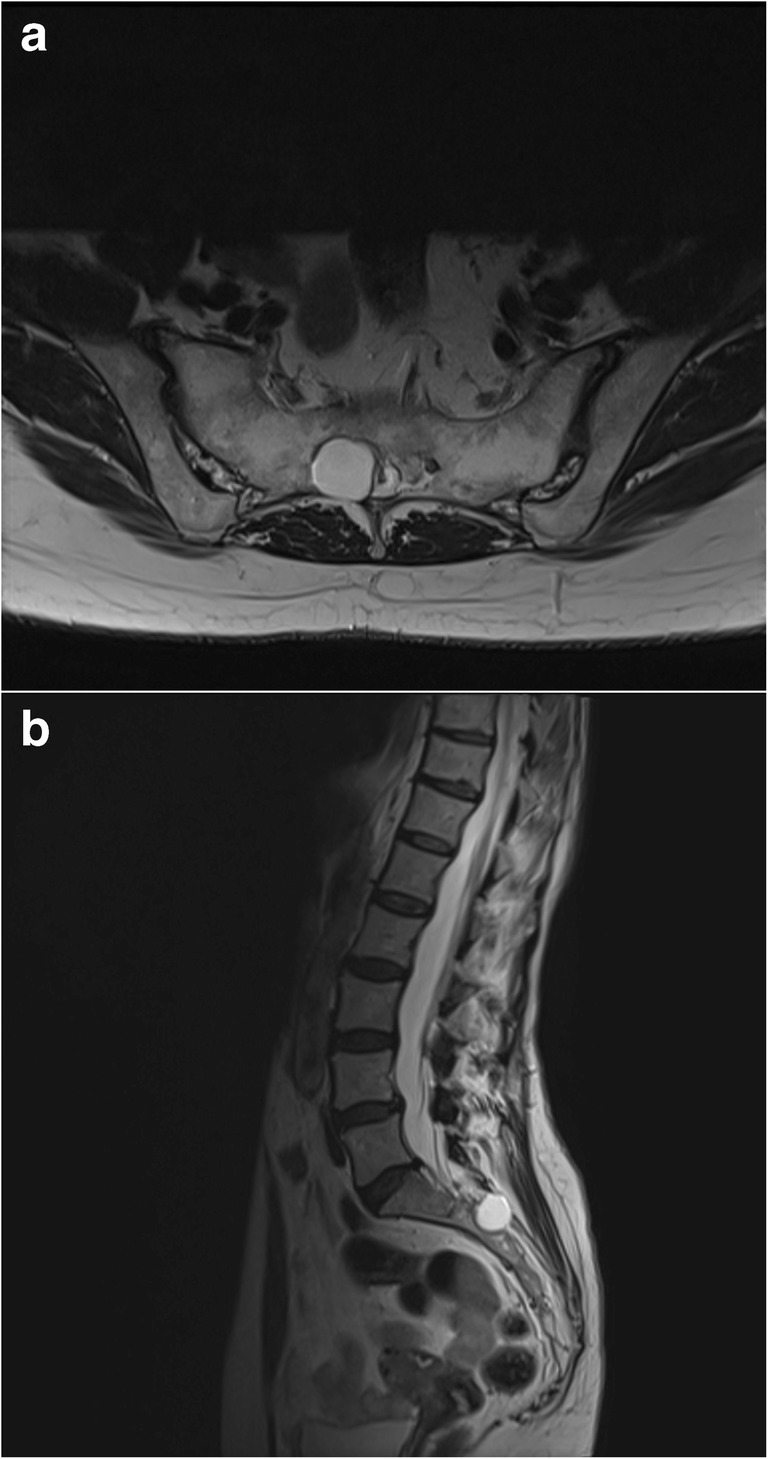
Axial (a) and sagittal (b) T2-weighted magnetic resonance images showing a sacral perineural cyst
